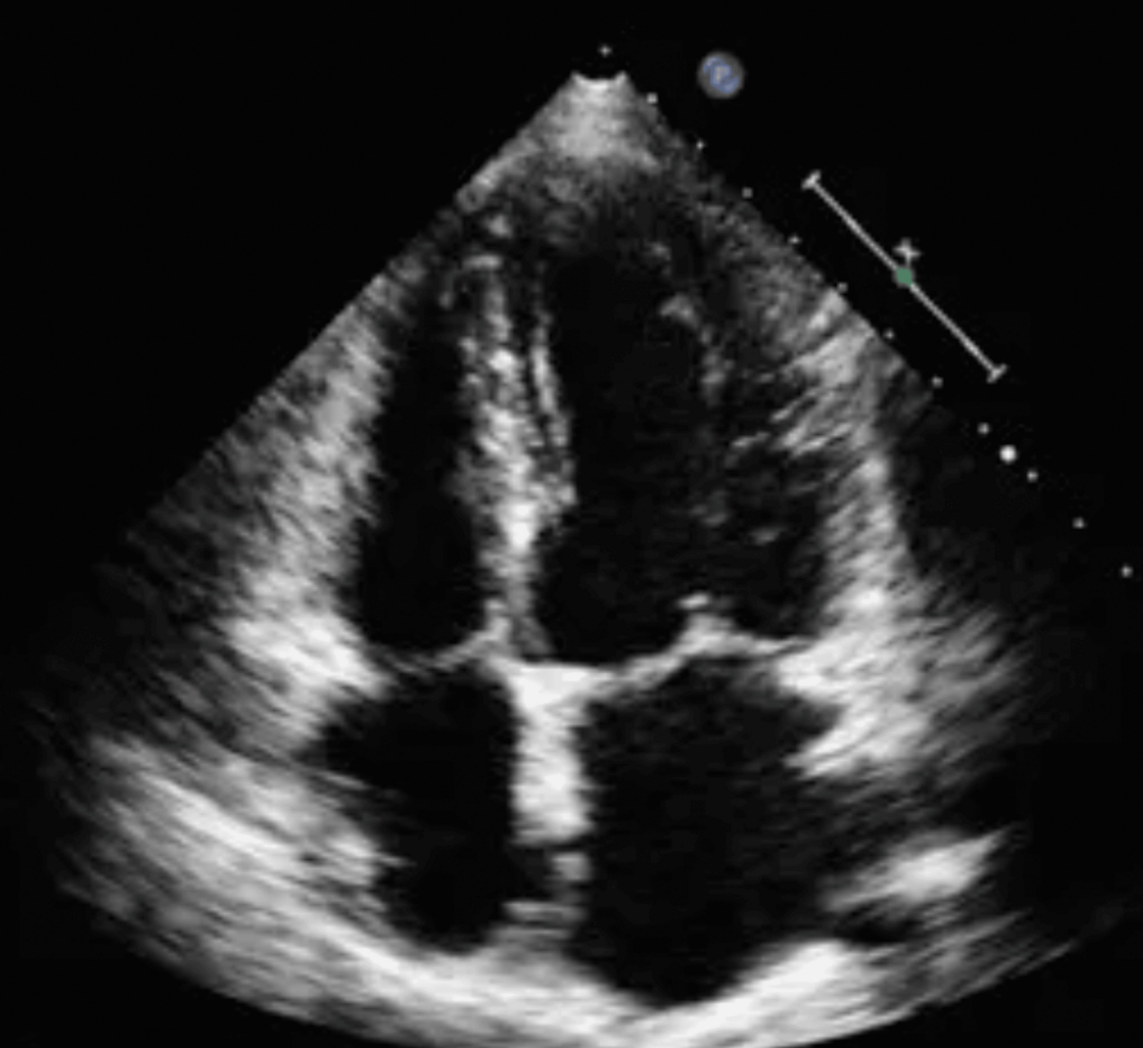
Echocardiogram displaying no pathological findings, including no vegetations or pericardial effusions, supporting the notion that the source of infection is not cardiac.
